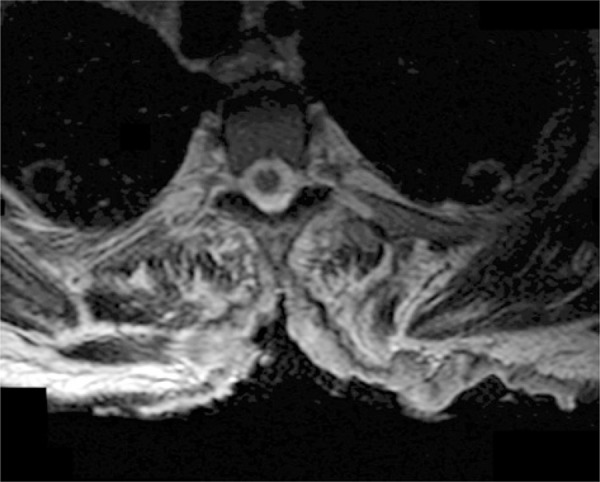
Axial view of the spine at T5 using magnetic resonance imaging demonstrating absence of spinal cord involvement
